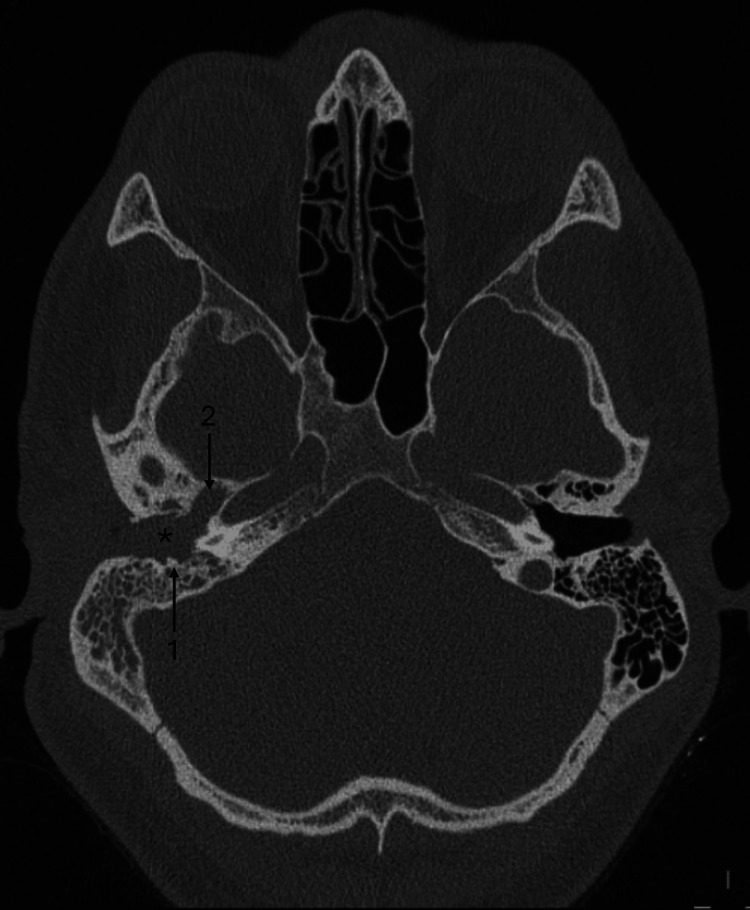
CT temporal bones CT temporal bones demonstrating a right EAC mass (*) causing erosion of the EAC (1) and opacification of the middle ear (2).
